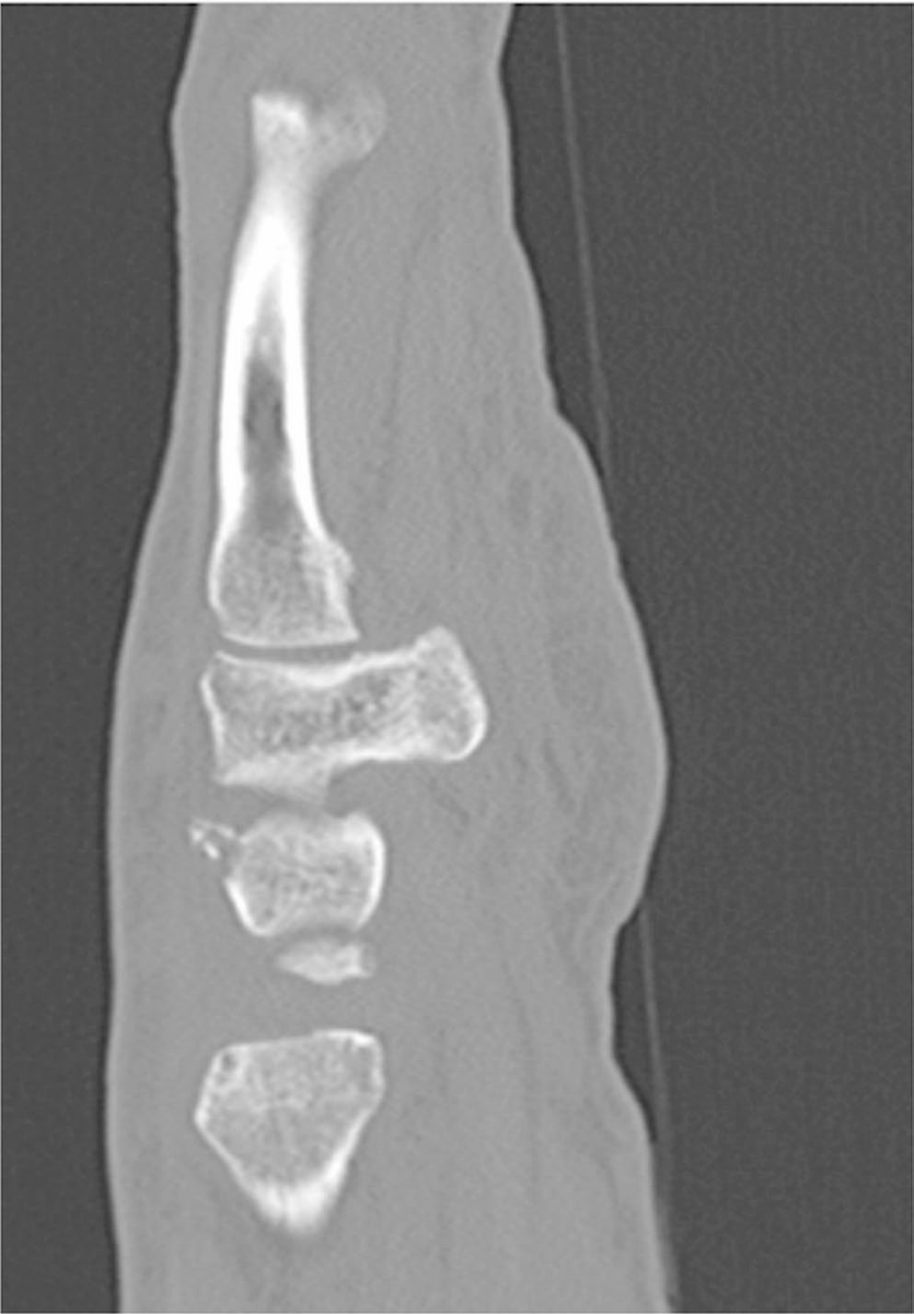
Typical CT scan findings in a sagittal reconstruction
